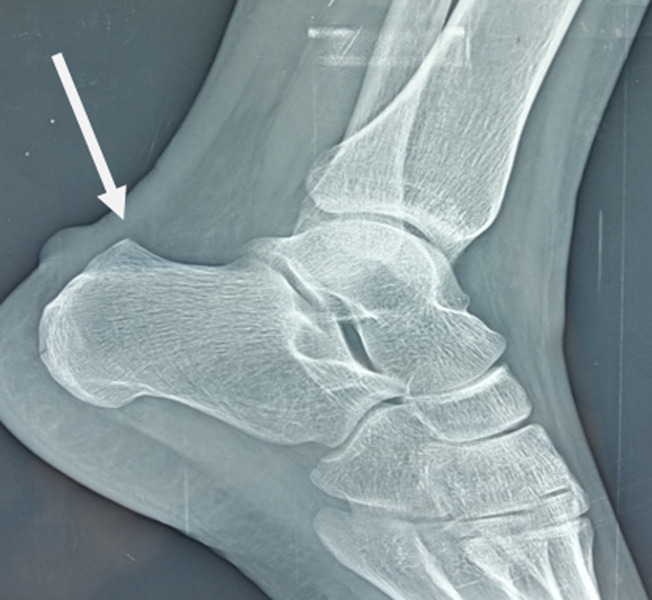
radiographie de profil du pied droit montrant la maladie de Haglund
